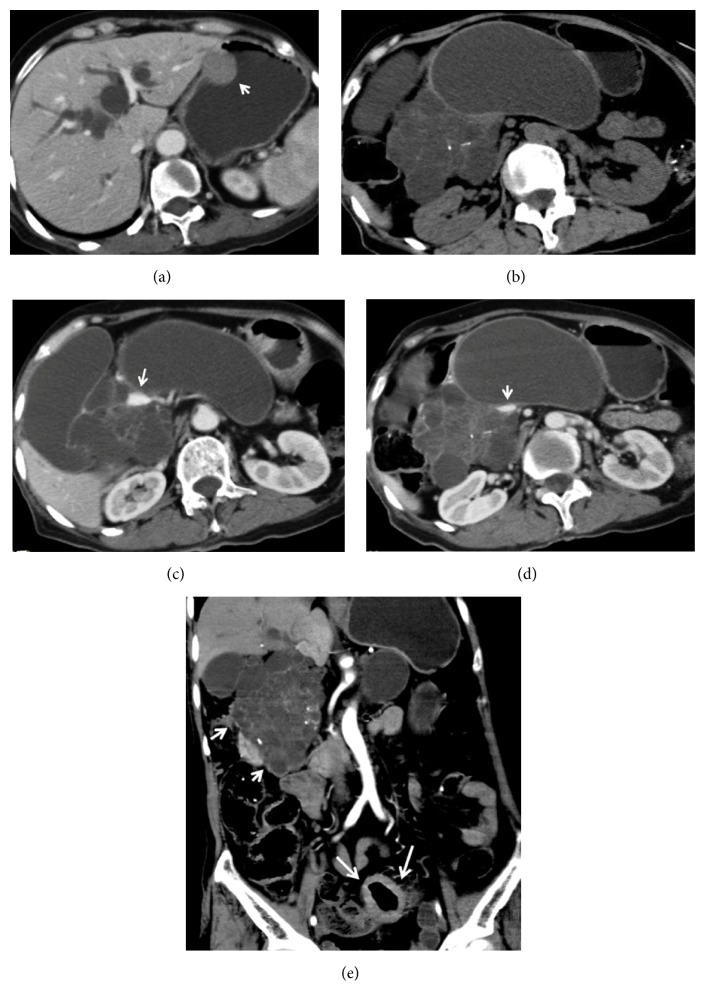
A 68-year-old female patient with a giant serous cystadenoma of the pancreatic head, concurrent colorectal cancer, and gastric stromal tumor. (a) Intrahepatic bile duct dilation and a gastric stromal tumor (arrow) were detected on CT plain scanning. (b) The pancreatic head tumor showed honeycomb-like appearance with multiple macrocysts and punctuate calcification. ((c) and (d)) Encasement of the portal and superior mesenteric veins (arrow) was noted on portal phase image. (e) The coronal CT image showed a pancreatic head tumor (short arrow) and rectal mass (long arrow).
